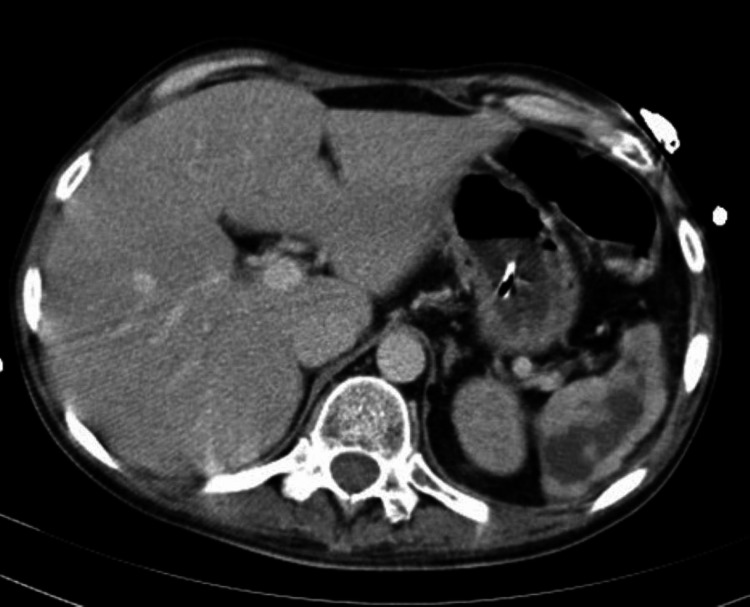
Abdominal cut of the full body CT scan Reveals the presence of nonspecific splenic heterogeneity that could be related to vascular compromise
